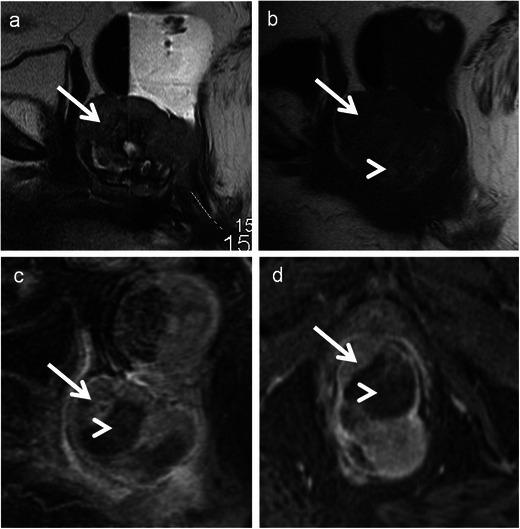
Rectal GIST in a 62-year-old man presenting with pain and rectal bleeding. a Sagittal T2-weighted MRI reveals a heterogeneous isointense to hypointense mass (*arrow*) arising from the anterior wall of the rectum and projecting into the rectoprostatic space. b Sagittal T1-weighted MRI reveals the lesion to be isointense on T1-weighted MRI with a few areas of hyperintensity (*arrowheads*) representing haemorrhage. c Sagittal and d axial contrast-enhanced MRI demonstrates intense enhancement (*arrow*) with central areas of non-enhancement (*arrowhead*) representing necrosis
